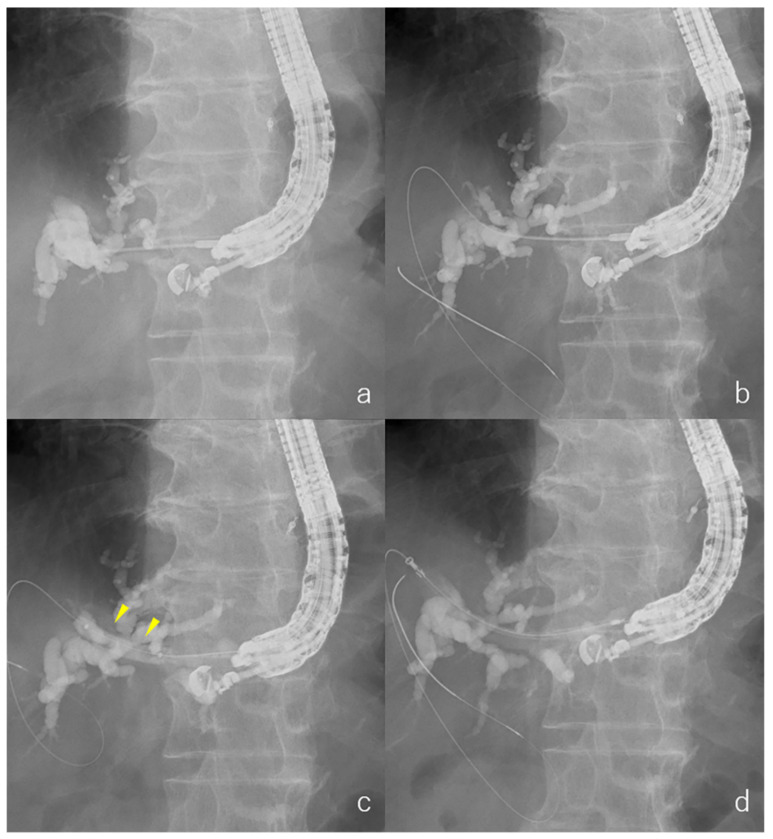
Radiographic images. (a) Puncture the bile duct; (b) After aspirating bile juice or contrast was used to confirm that it was a bile duct, a guidewire was inserted into the bile duct; (c) Tract dilation of a fine-gauge balloon catheter. (d) An SEMS was placed.
